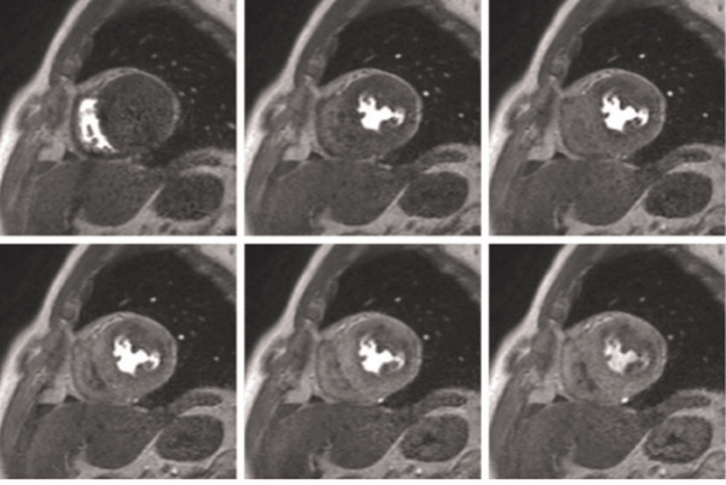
Stress perfusion MRI in the same patient with triple vessel disease. Subendocardial ischemia is clearly demonstrated in the anteroseptal well, lateral wall and inferior wall on high resolution images.
